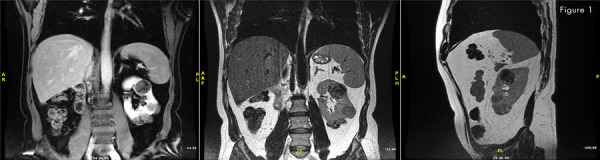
MRI report - solitary left kidney - at least eleven renal tumors.
